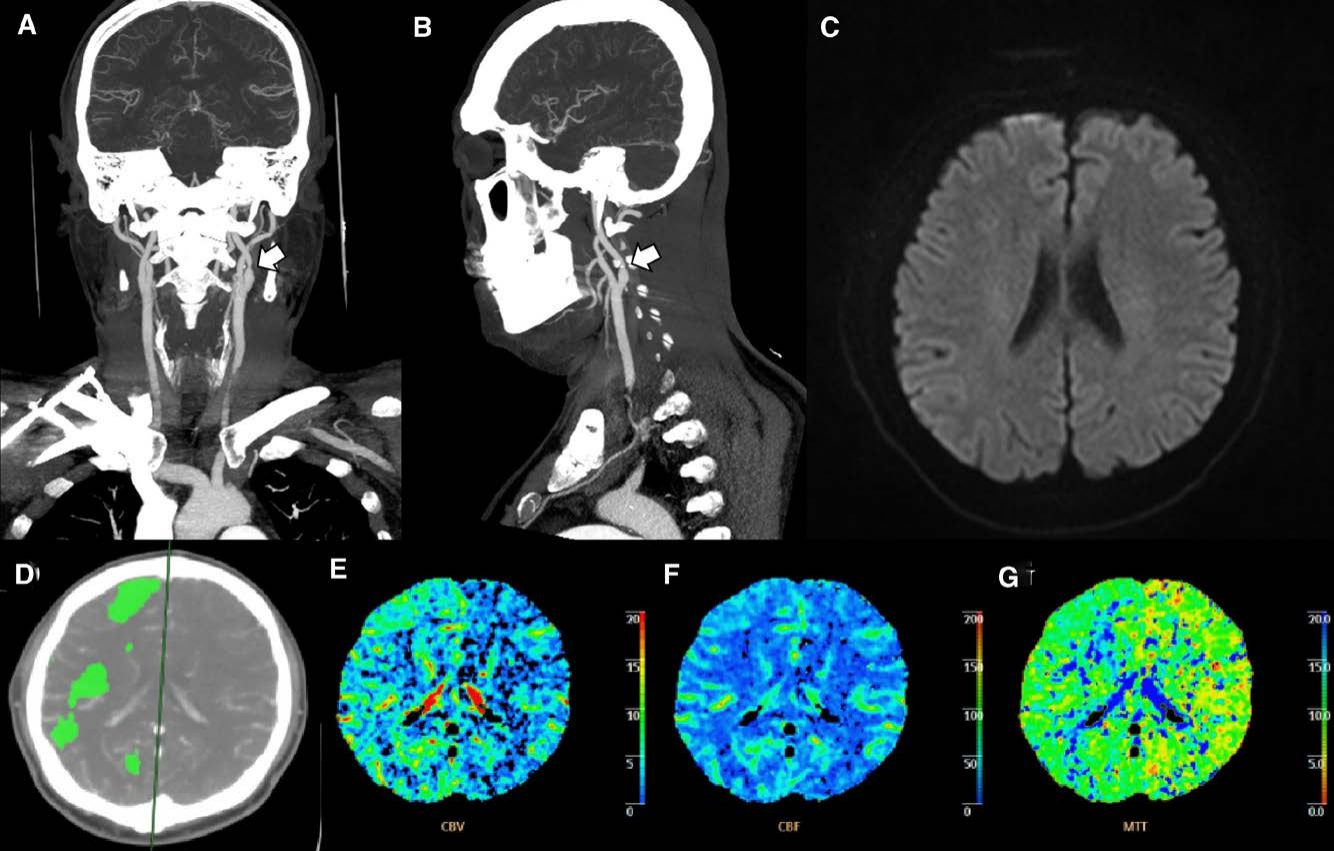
Transient right hemiparesis with left internal carotid artery (ICA) stenosis (Case 1). (A) Left ICA stenosis (arrow) in coronal computed tomography angiography (CTA) imaging. (B) Left ICA stenosis (arrow) in sagittal CTA imaging. (C) No restriction on diffusion-weighted magnetic resonance imaging. (D–G) Perfusion CTA images show similar cerebral blood flow on both sides but increased mean transit time and cerebral blood volume on the right hemisphere.
